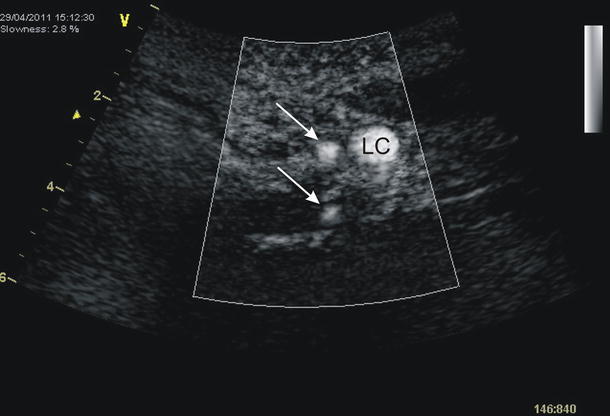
Duplication of the left vertebral artery in Doppler ultrasonography (B-flow mode): *arrows* duplicated left vertebral arteries, *LC* left common carotid artery
